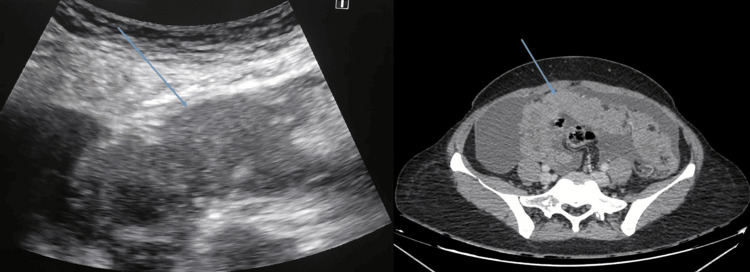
Ultrasound image and CT scan of one of the patients Ultrasound image showing omental thickening (blue arrow). CT scan abdomen of the same patient showing omental thickening and ascites (blue arrow).
